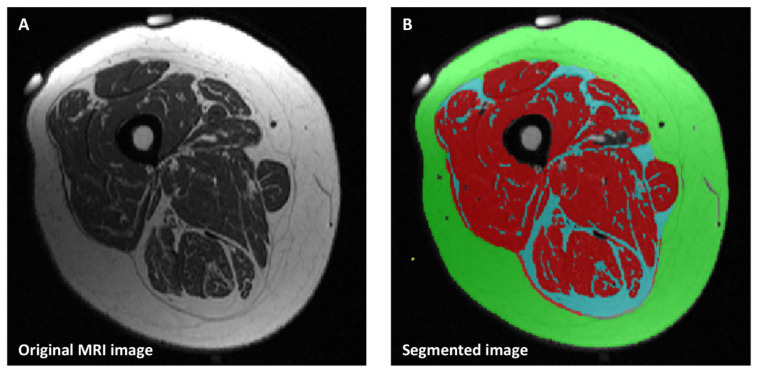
A sample of single-slice mid-thigh MRI image before (A) and after (B) segmentation. Structures in red: muscle, green: subcutaneous fat, blue: intermuscular fat.
